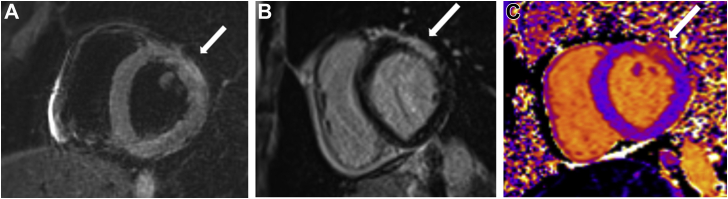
Cardiac magnetic resonance imaging showing (A) a spontaneous hypersignal T2 in the anterior and lateral territory in favour of edema, (B) anterolateral subepicardial enhancement on late gadolinium enhancement imaging, and (C) T1 mapping image in the same territory suggesting cardiomyocytic necrosis.
